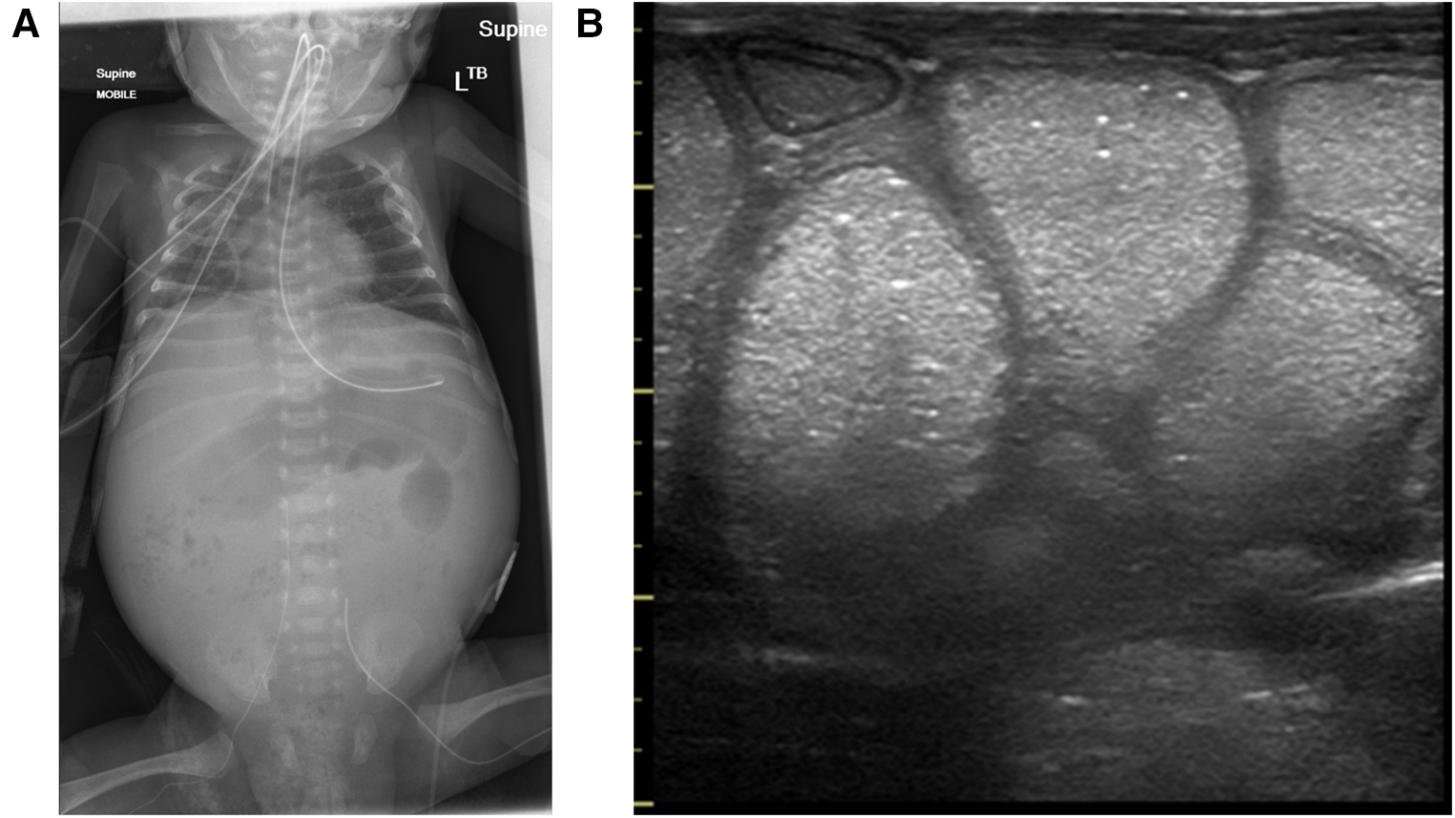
Milk-curd obstruction concurrent x-ray and point-of-care abdominal ultrasound findings. (A) Plain abdominal x-ray showing paucity of bowel gas with a soap-bubbly appearance on the right. (B) The sonographic appearance of the bowel showing islets of echogenic homogenous material within the lumen maintaining the normal bowel-wall sonographic appearance.
